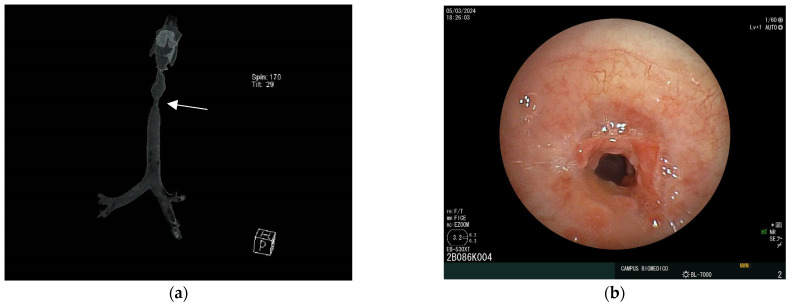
Benign subglottic stenosis secondary to traumatic intubation treated by sequential rigid dilations. (a) Reconstruction of CT scan of trachea showing mid-tracheal stenosis (arrow pointing at narrowest caliber); (b) bronchoscopic images showing post intubation scar with stenosis of tracheal lumen.
